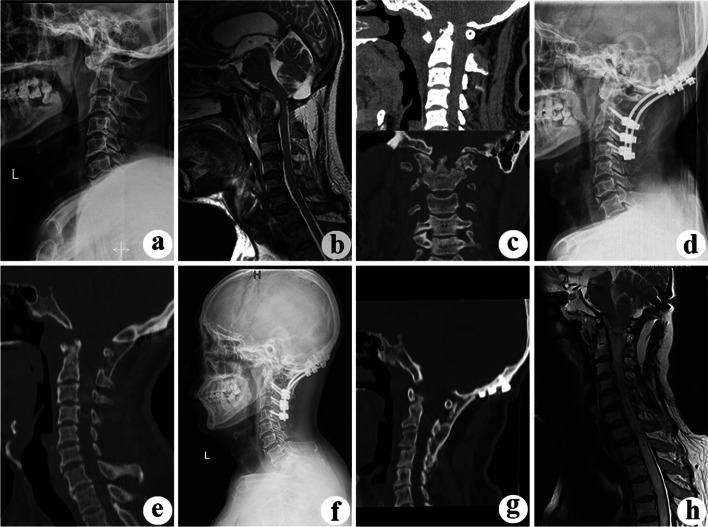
A 44-year-old man with upper cervical spine TB was treated using endoscopy-assisted anterior cervical debridement combined with posterior fixation and fusion. A preoperative lateral X-ray film (a), MRI (b), and CT (c) show C1/2 vertebral destruction, spinal cord compression, and abscess formation. A postoperative X-ray (d) shows satisfactory posterior occipital-cervical fixation and a well-corrected cervical vertebral sequence. CT (e) shows that the bone block is well placed. At the final follow-up at 60 months postoperatively, a radiograph (f) shows well-placed fixation, and CT (g) shows solid bony union. MRI (h) shows no abscess formation and no spinal cord compression
